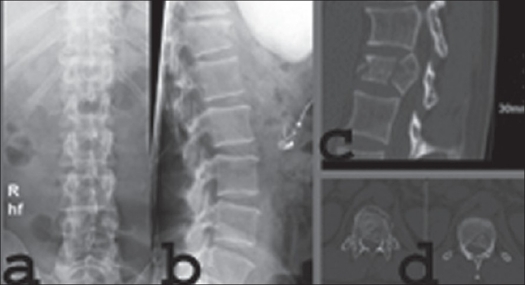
Plain x-ray AP (a) and lateral (b) sagittal reconstructioin CT (c) and axial CT scan (d) Spinal shows burst fracture L1 vertebrae with canal compromise
